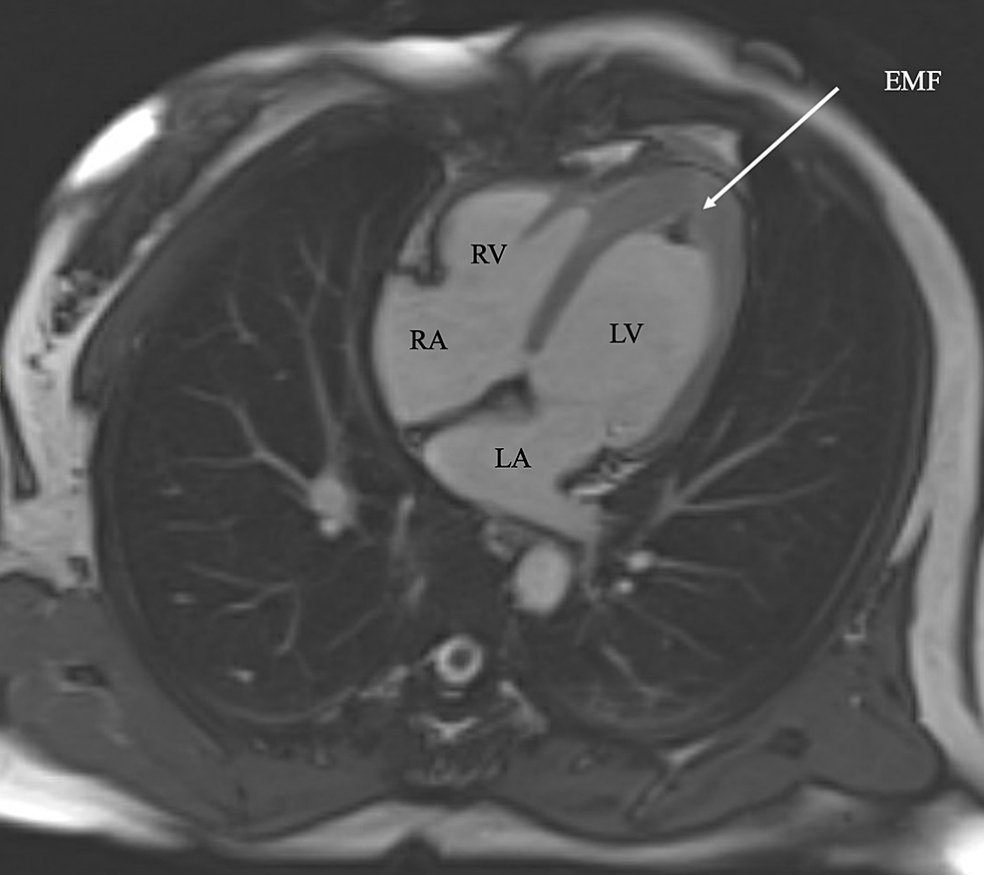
cMRI four-chamber view depicting endomyocardial fibrosis after the completion of a year-long course of anticoagulation with apixaban for LV thrombus. cMRI - cardiac magnetic resonance imaging, EMF - Endomyocardial fibrosis
